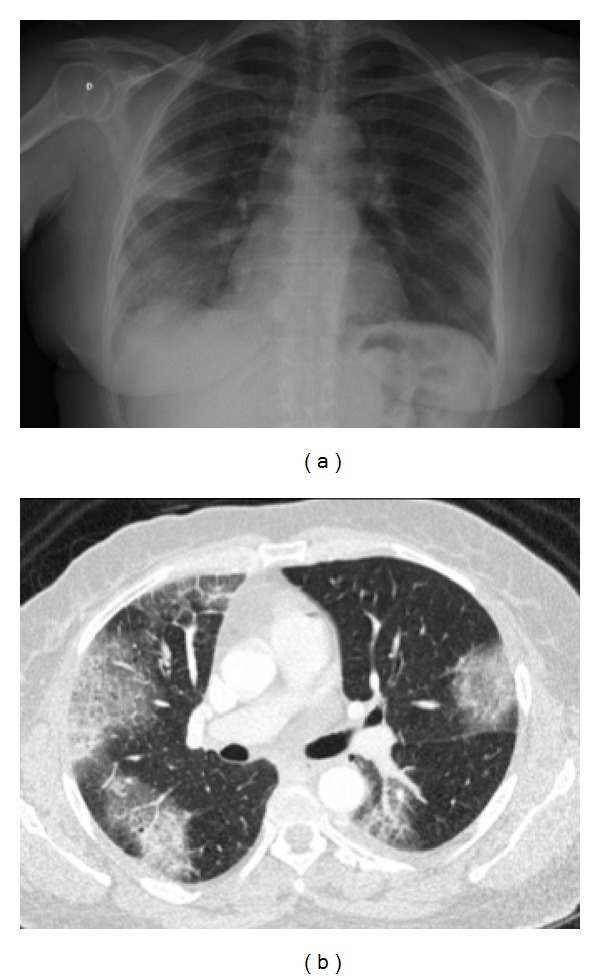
Chest X-ray and tomography*: chest X-ray revealed ill-defined opacities in both lungs, especially in the middle and inferior field of the right lung (a). There was no mediastinal enlargement, a remarkable sarcoidosis radiographic sign. Axial high-resolution computer tomography (HRCT) scan revealed multiple ground-grass opacities in bilateral subpleural areas, associated with interlobular septal thickening (b).
